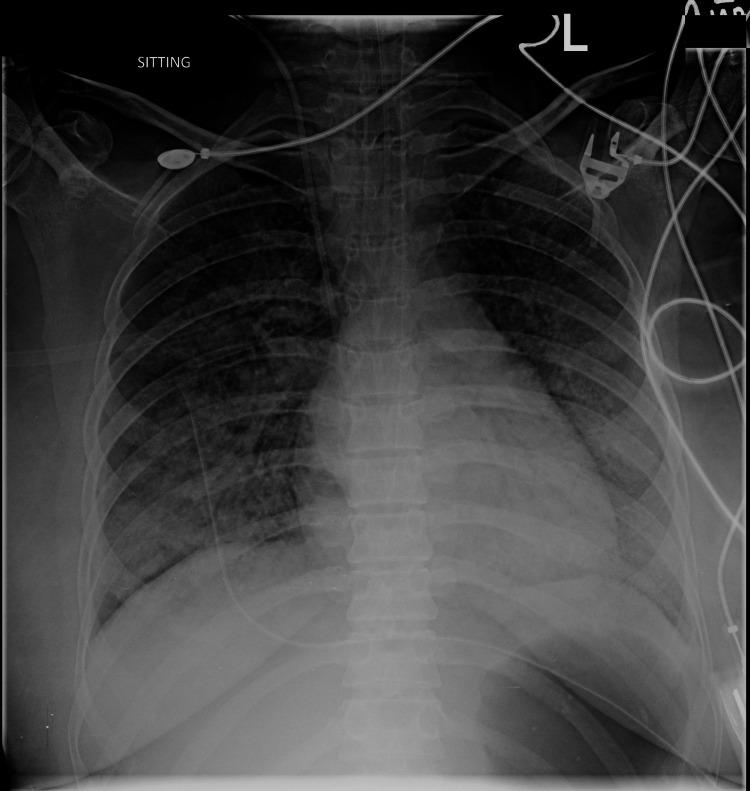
Chest x-ray (anteroposterior view) after resuscitation
